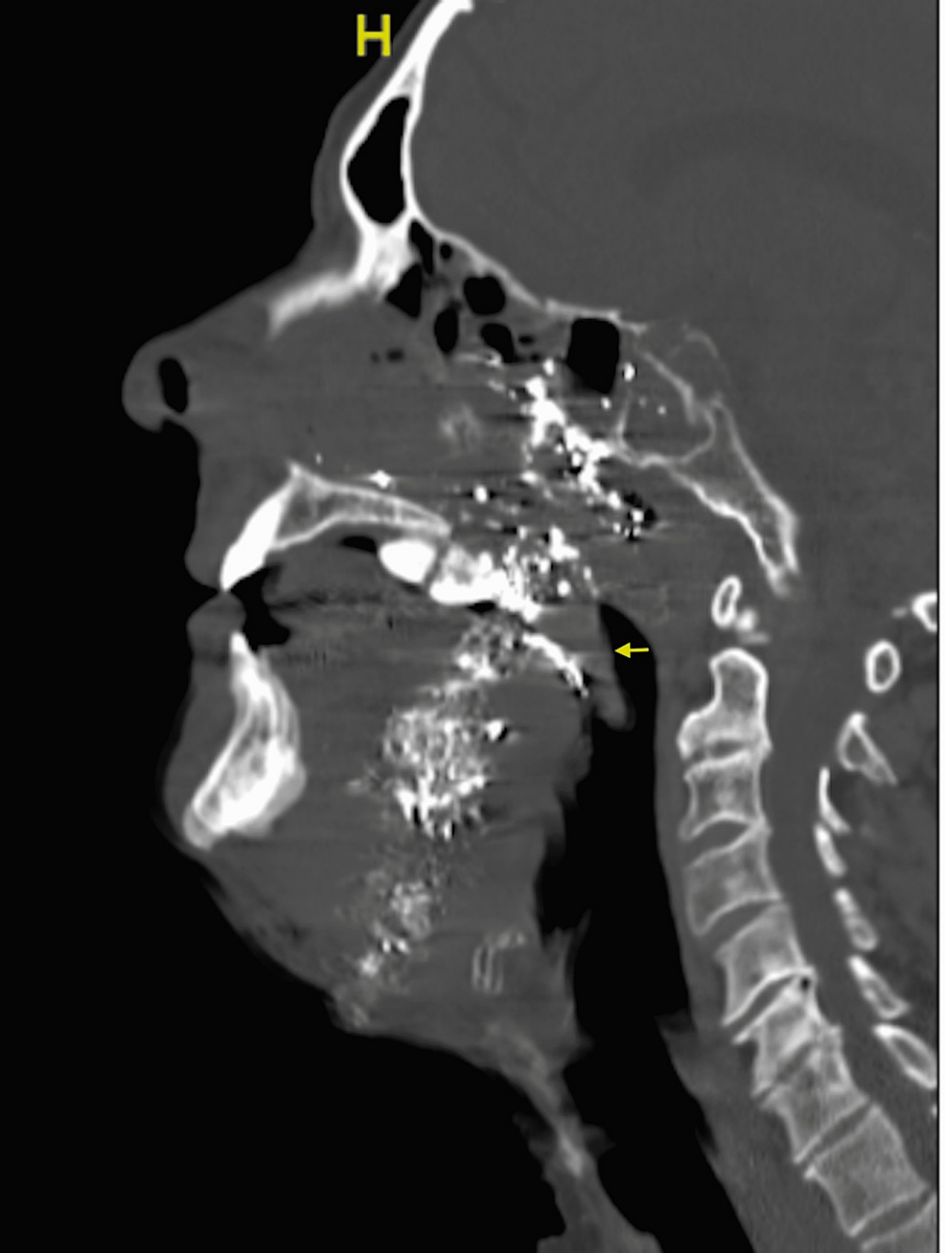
CT face without intravenous contrast with a sagittal view. Hard palate fracture (yellow arrow) and multiple bullet fragments at the sphenoid sinuses, ethmoid air cells, nasal cavity, posterior nasopharynx mucosa, and hard palate.
